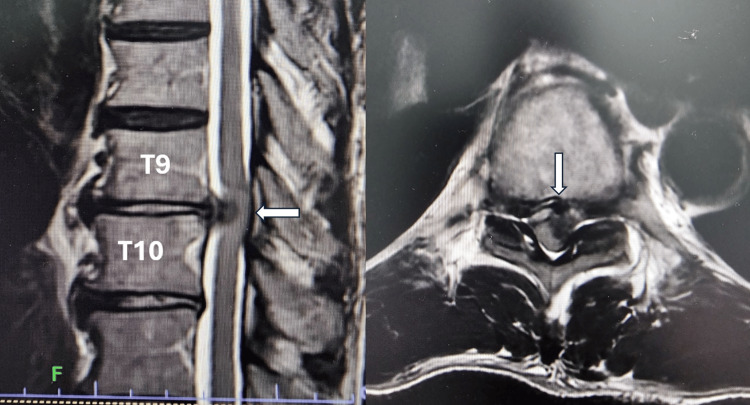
MRI demonstrated a large paracentral disc at T9/T10 compressing the spinal cord.
